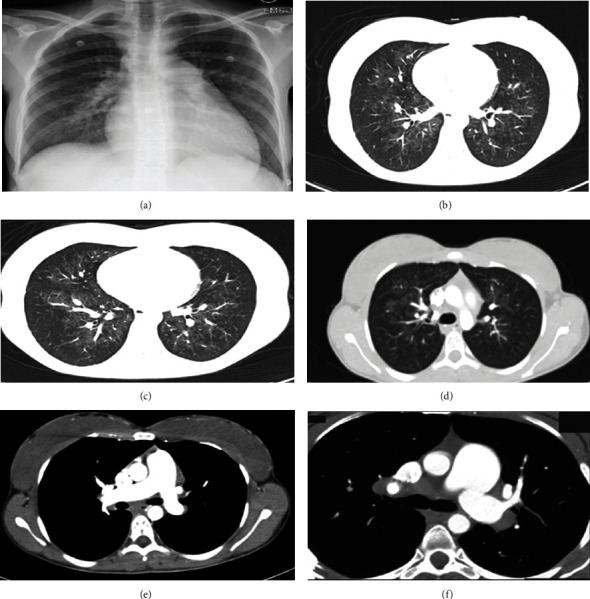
Admission CXR (a) showing clear lung fields and normal cardiac silhouette. High-resolution CT chest scan (b–f) showing bilateral peri-bronchovascular ground glass densities involving upper and lower lobes with smooth interlobar septal thickening, mediastinal lymphadenopathy, and pulmonary trunk dilatation.
